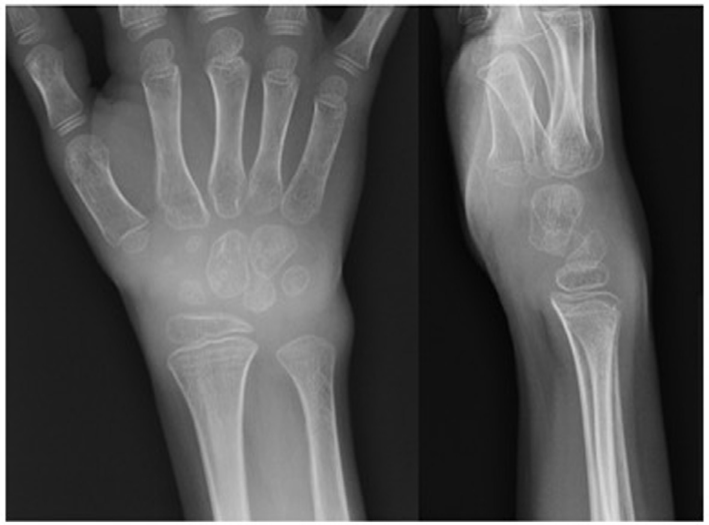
Right wrist radiograph. Radiographic changes consistent with damage secondary to inflammatory arthritis were observed. The patient had approximately a 6-month history of a decreased range of motion of right wrist. Widening of the scapholunate interval is noted on the AP view. Volar displacement of the capitate from the axis of the radius and lunate and volar rotation of the lunate are seen on the lateral view. Periarticular osteopenia and soft tissue swelling are seen on both views.
